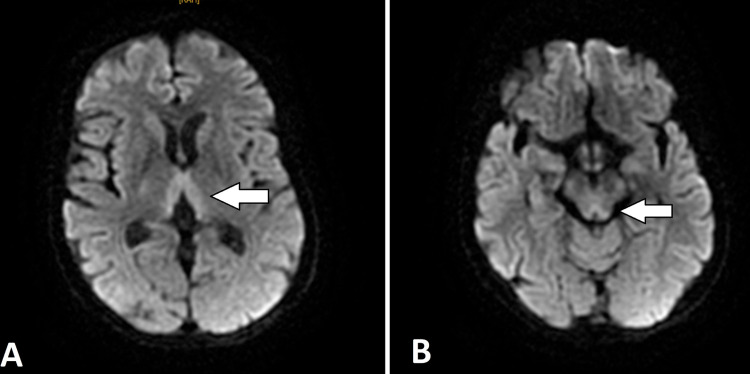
Axial sections of brain MRI in diffusion sequence B1000 Hyper signal abnormalities on medial thalamus (A) and the mammillary bodies (B).
